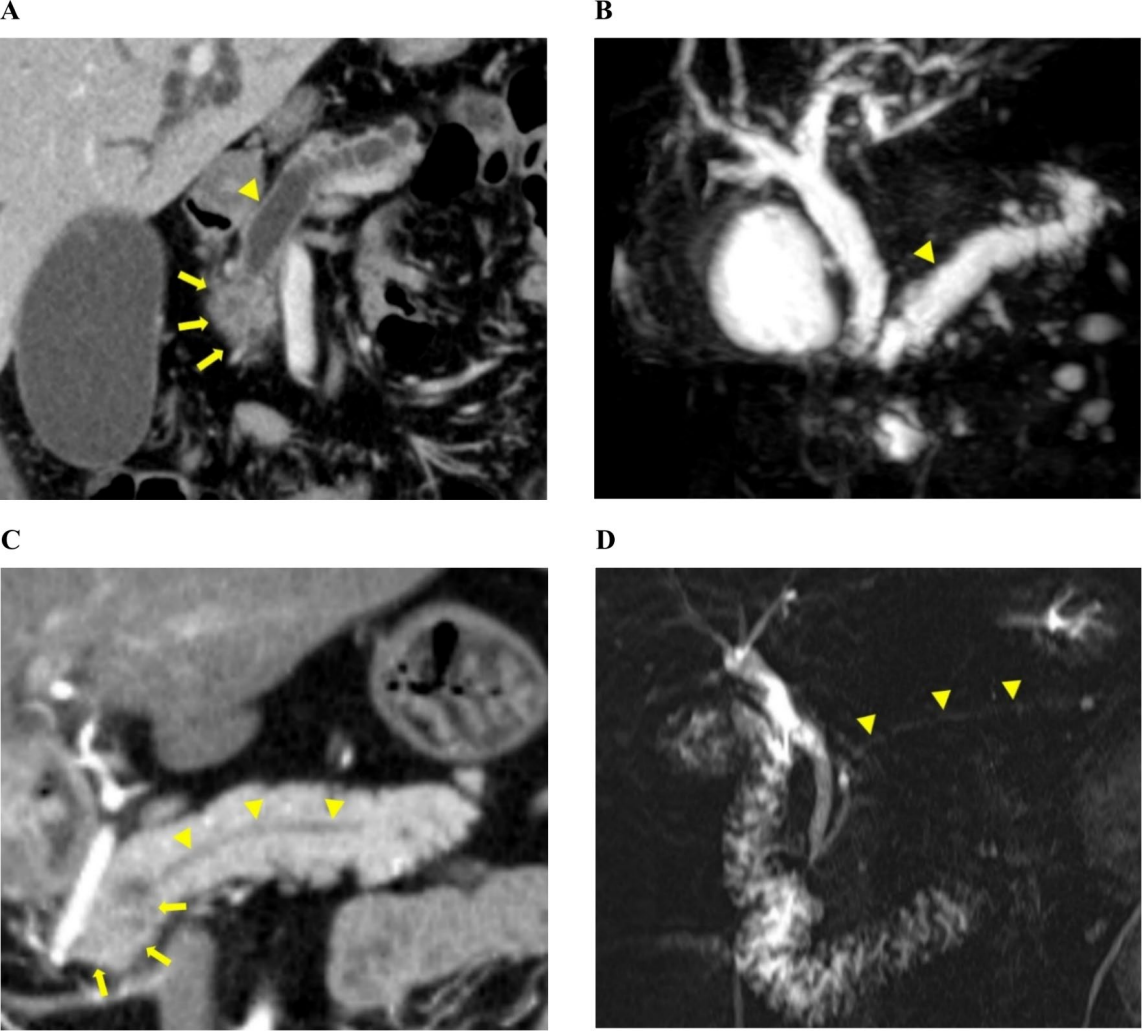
Representative images of cases with and without pancreatic ductal occlusion. A and B CT (A) and MRCP images (B) of a 69-year-old man with pancreatic ductal occlusion showing pancreatic cancer (arrows) and a dilated main pancreatic duct (arrowhead). C and D, CT (C) and MRCP images (D) of a 73-year-old man without pancreatic ductal occlusion showing pancreatic cancer (arrows) and an undilated main pancreatic duct (arrowhead). *CT* computed tomography, *MRCP* magnetic resonance cholangiopancreatography
